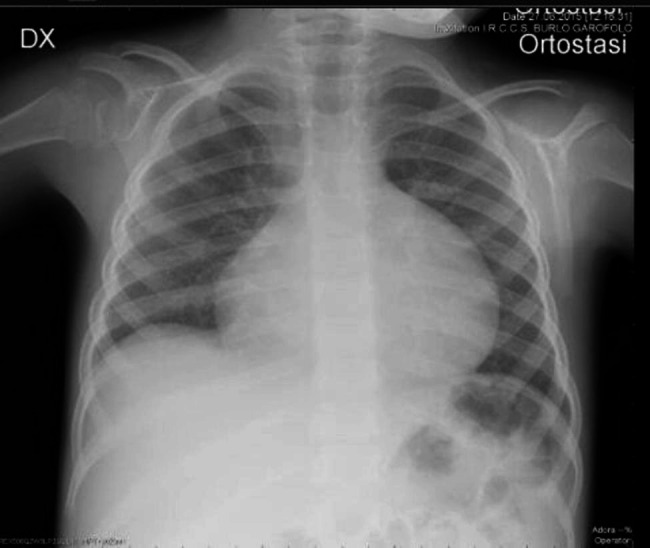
Chest x-ray: flask appearance of the cardiac shadow due to pericardial effusion
